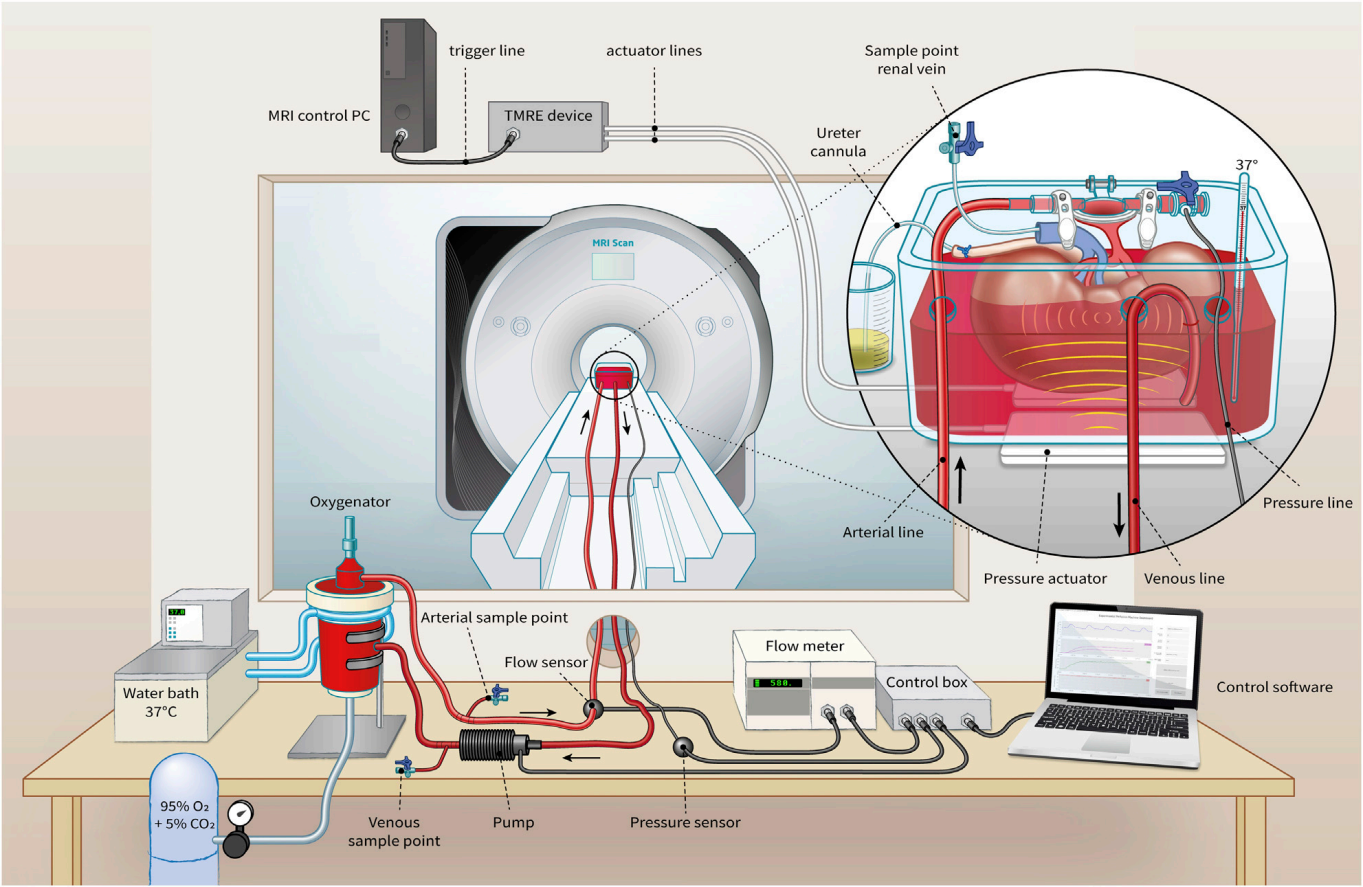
Representation of MRI-compatible NMP setup for *ex vivo* kidney perfusion including Tomoelastography setup. Pressure actuators induce compression wave (yellow) to the organ chamber, evoking shear waves (light red) in the kidney.
